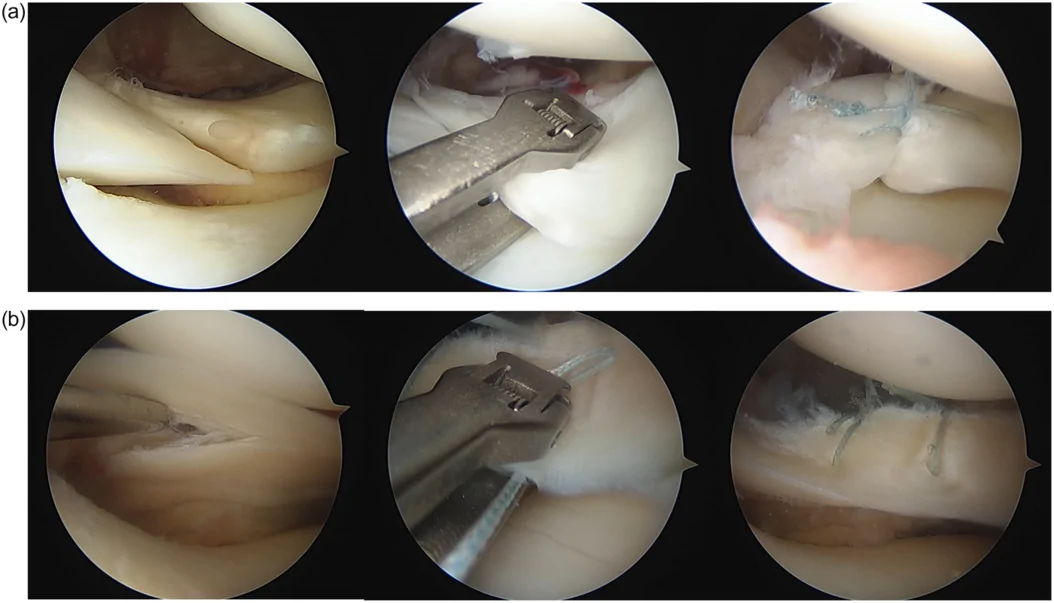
Arthroscopic findings of lateral meniscus oblique radial tear (LMORT) and longitudinal tears. (a) LMORT of the posterior horn of the lateral meniscus. Left: oblique radial disruption of the circumferential fibres. Middle: side‐to‐side repair using a suture passer. Right: appearance after side‐to‐side repair. (b) Posterior longitudinal tear of the lateral meniscus. Left: longitudinal tear of the posterior horn. Middle: all‐inside repair using a suture passer. Right: appearance after all‐inside repair. Arthroscopic images were obtained with the knee in the figure‐of‐four position under fluid distension, which opens the lateral compartment; therefore, they do not represent resting meniscal position. Extrusion was measured on MRI. MRI, magnetic resonance imaging.
